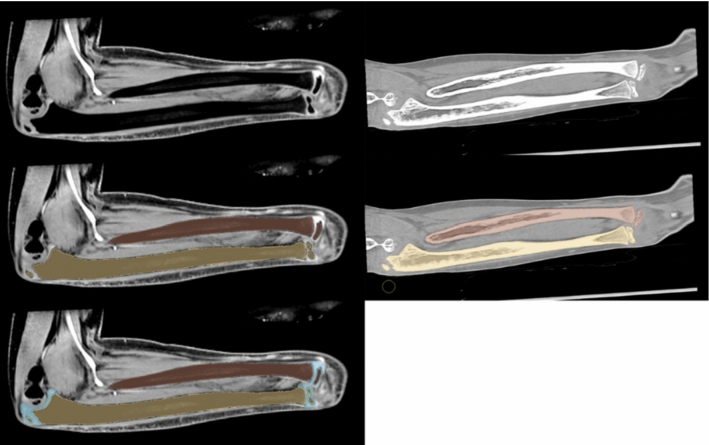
Top row: example of the MRI (left) and CT (right) of lower right arm. Middle row: MRI and CT overlaid with radius (brown) and ulna (yellow) bone segmentation. Bottom row: MRI overlaid with bone and cartilage (blue) segmentation
